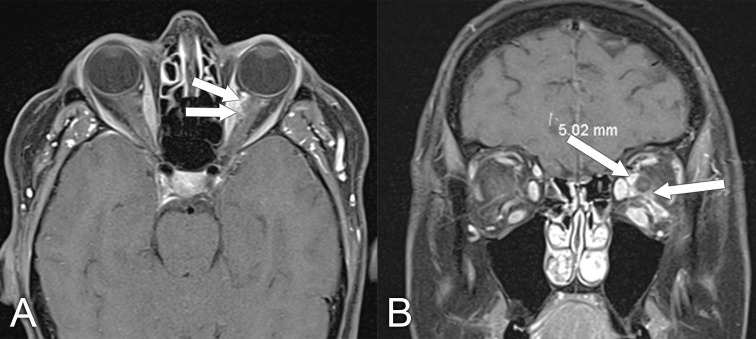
MRI findings in a 59-year-old woman with a history of metastatic breast cancer. For the past 3 months, she has noticed new-onset binocular diplopia. On clinical exam, she has mild left enophthalmos and restricted left eye movements. (A) Axial T1 postcontrast MRI with fat saturation shows enlargement of the left medial rectus muscle belly, as well as an intraconal infiltrative process in the left orbit (arrows). (B) Coronal T1 postcontrast MRI with fat saturation confirms enlargement of both the medial and inferior rectus muscles and an intraconal component of the lesion (arrows). This lesion was biopsied and confirmed to be metastatic breast carcinoma.
